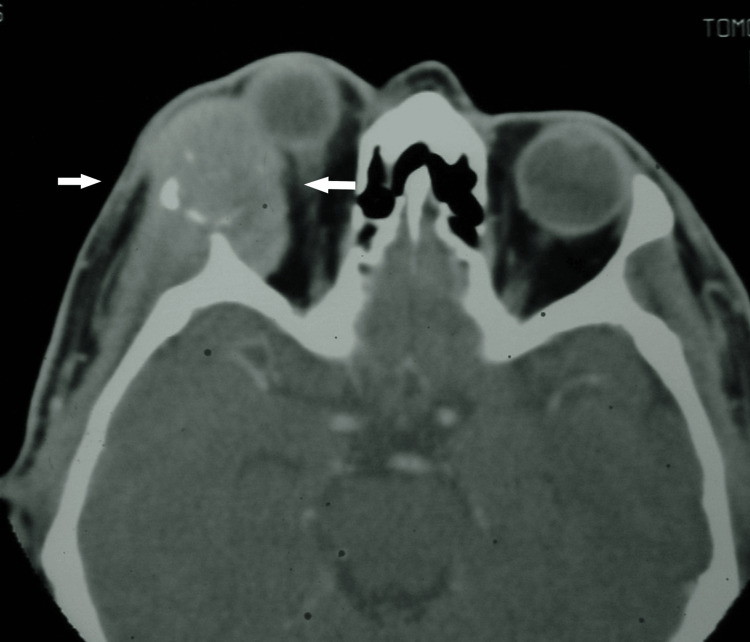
Axial computed tomography (CT) image demonstrating an enlarged lacrimal gland mass (white arrows) with erosion of the temporal bone and extension into the temporalis fossa.
